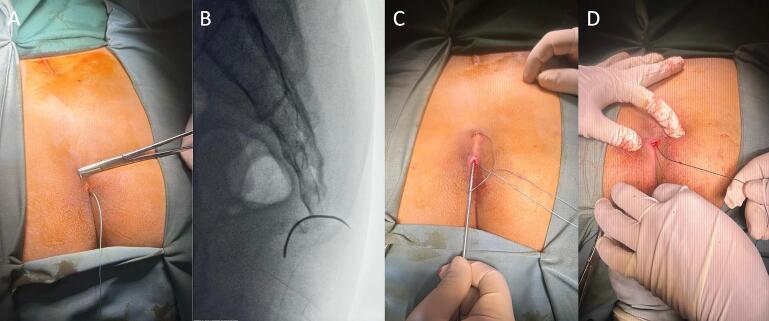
The patients under general anaesthesia are in the prone position. A minimal incision of about 1 cm was performed close below the fracture site; then, the initial suture was passed. (a) A lateral X-ray view showed a needle connecting the sacrum and coccygeal using intraosseous procedures. (b) After the first suture, the thread was pulled back into the initial incision subcutaneously. (c) A minimal invasive figure of eight procedures was figured out, and the suture's end was tied (d).
